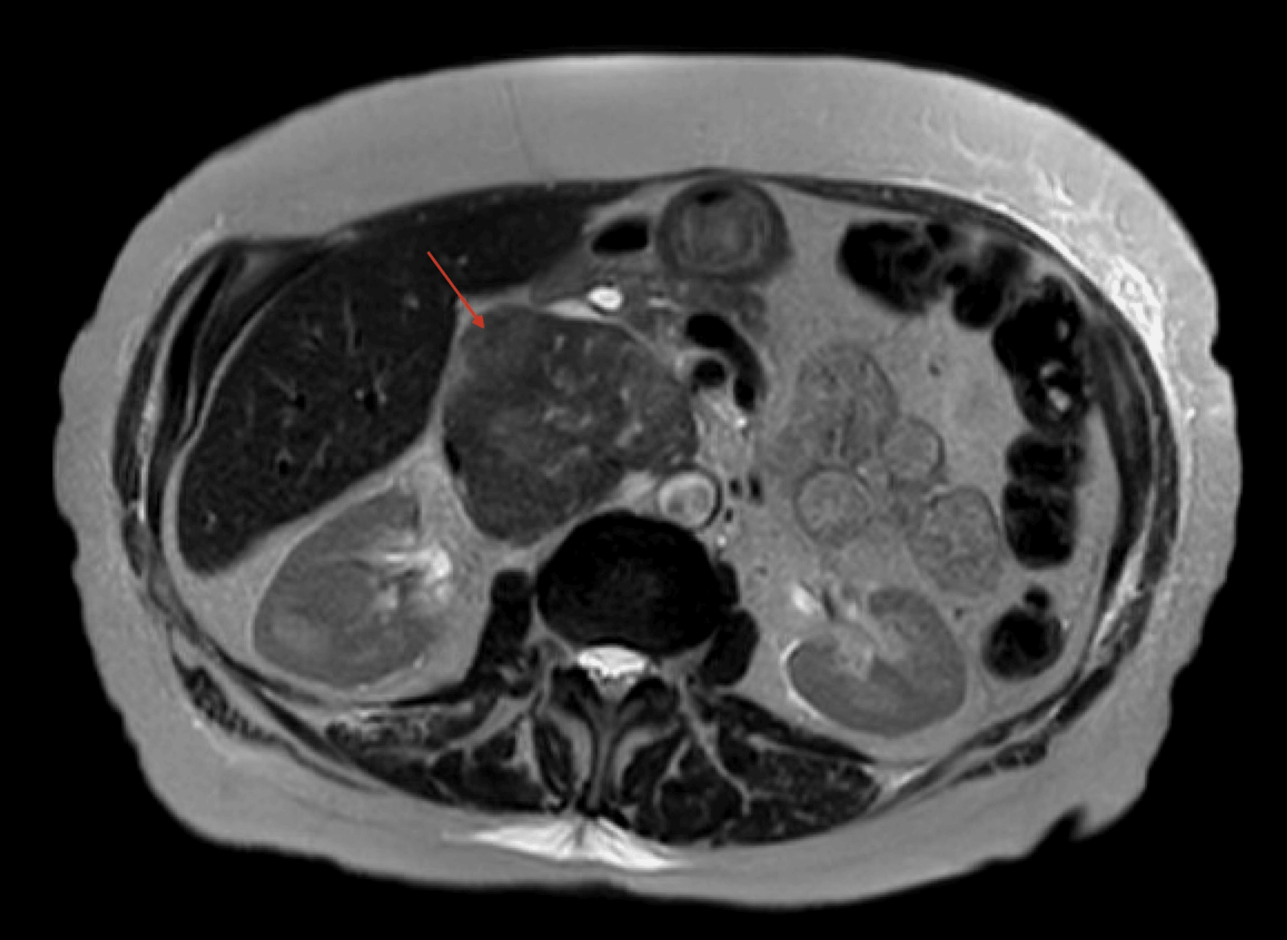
Follow-up MRI of the abdomen confirming a large right-sided retroperitoneal mass, possibly invading the inferior vena cava.
